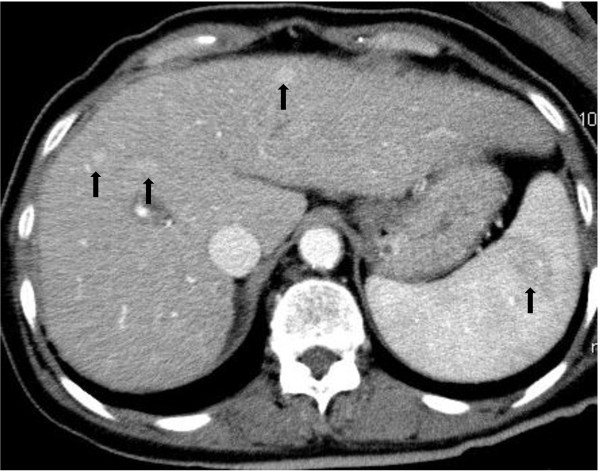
Contrast-enhanced computed tomography scan shows metastatic tumors in the liver and spleen (arrows).
